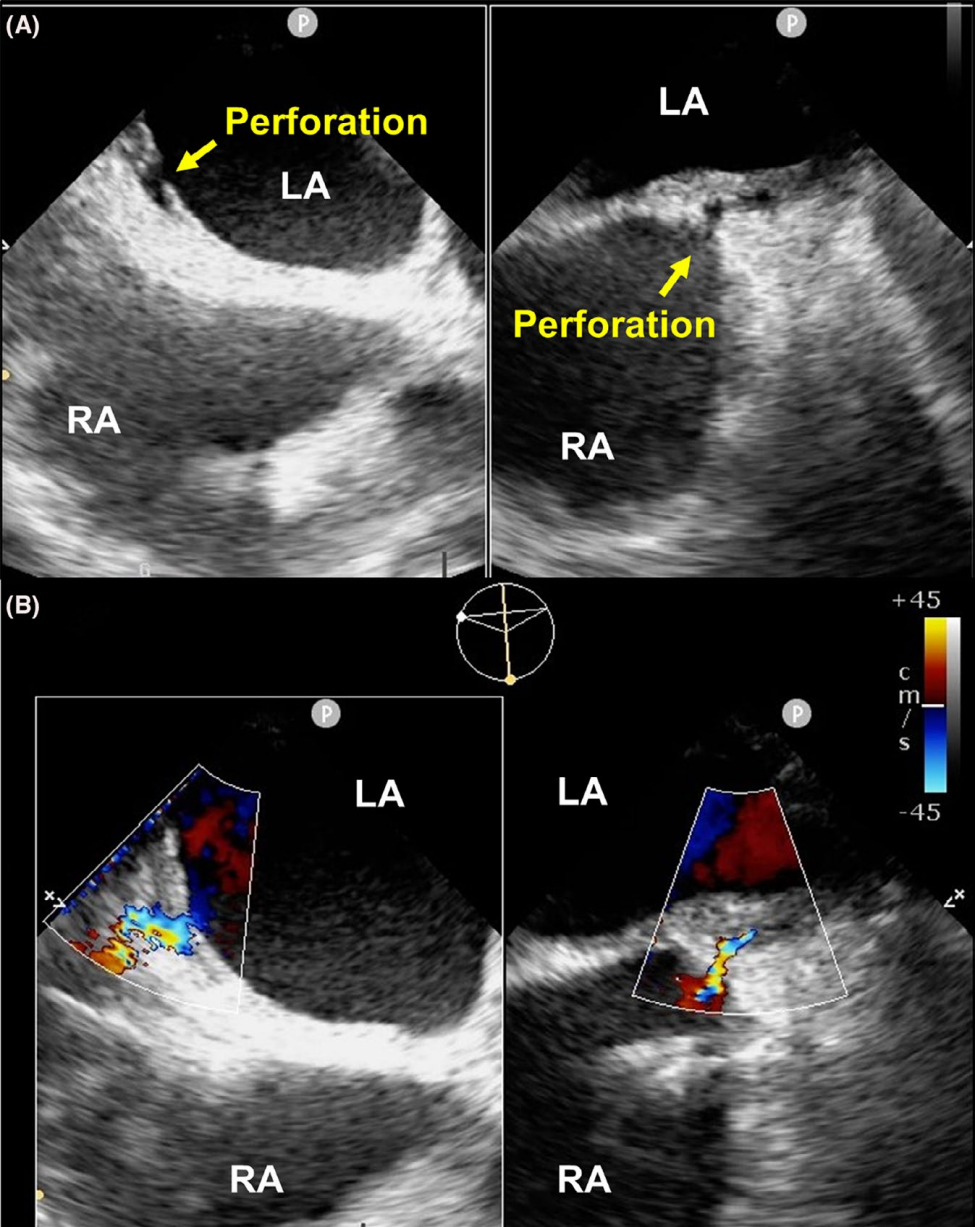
(A) A two‐dimensional image revealing the atrial septal perforation. (B) A color‐flow Doppler image revealing the atrial septal perforation. LA, left atrium; RA, right atrium
